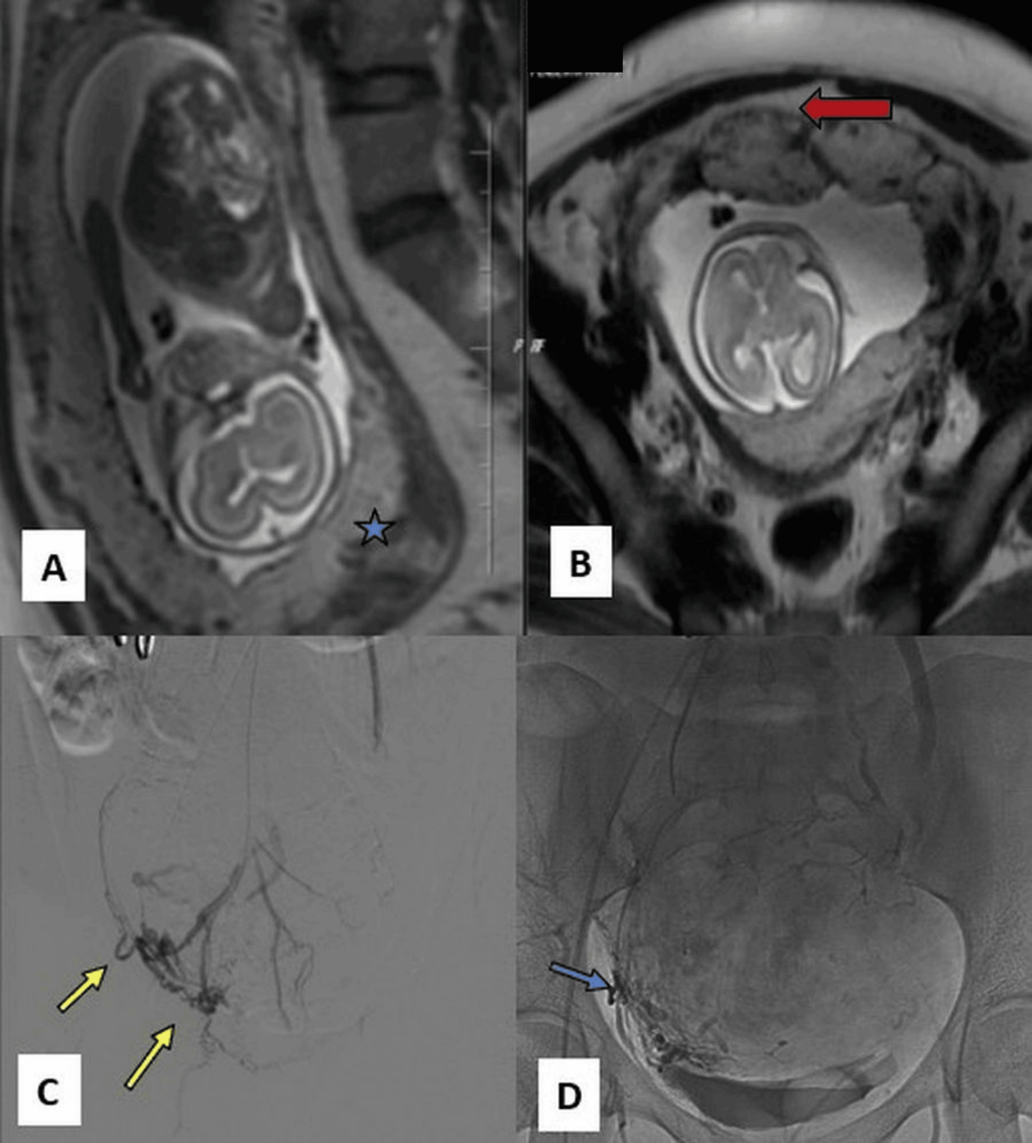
Magnetic resonance imaging of the pelvis with placenta and internal iliac angiogram: A, sagittal section; B, coronal section; C and D, right internal iliac angiogram. (A and B) Intrauterine foetal growth at 30 weeks with complete placenta previa and retroplacental haemorrhage. (A) A loss of interface between the decidua basalis and myometrium (blue star), with placental invasion into the serosa in the anterior layer (red arrow) (B). (C) Multiple tiny vesical feeders from the inferior and superior branches (yellow arrows) supplying the placenta percreta. (D) Post-embolisation images with an embolic cast within the placenta percreta branches (blue arrow).
